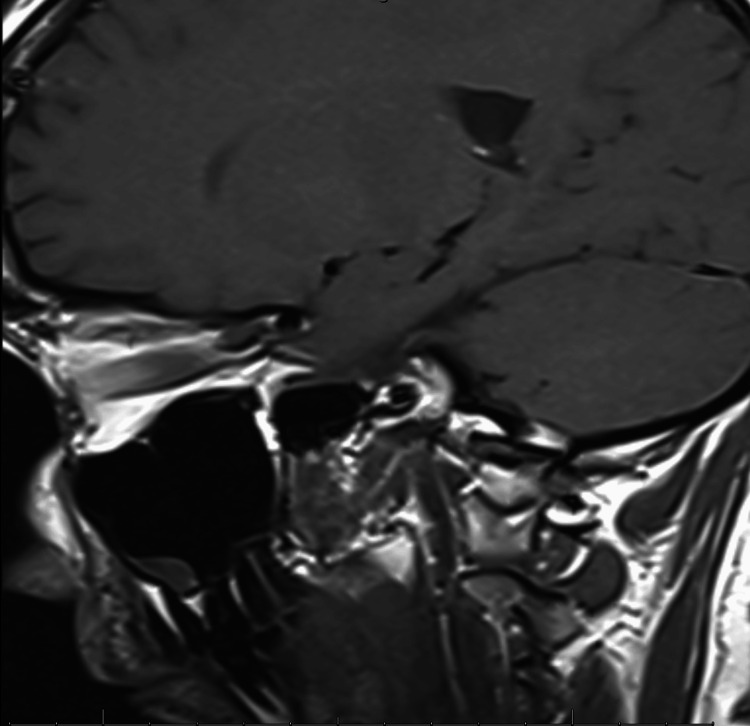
Sagittal view of brain MRI showing normal pituitary gland morphology MRI: magnetic resonance imaging
